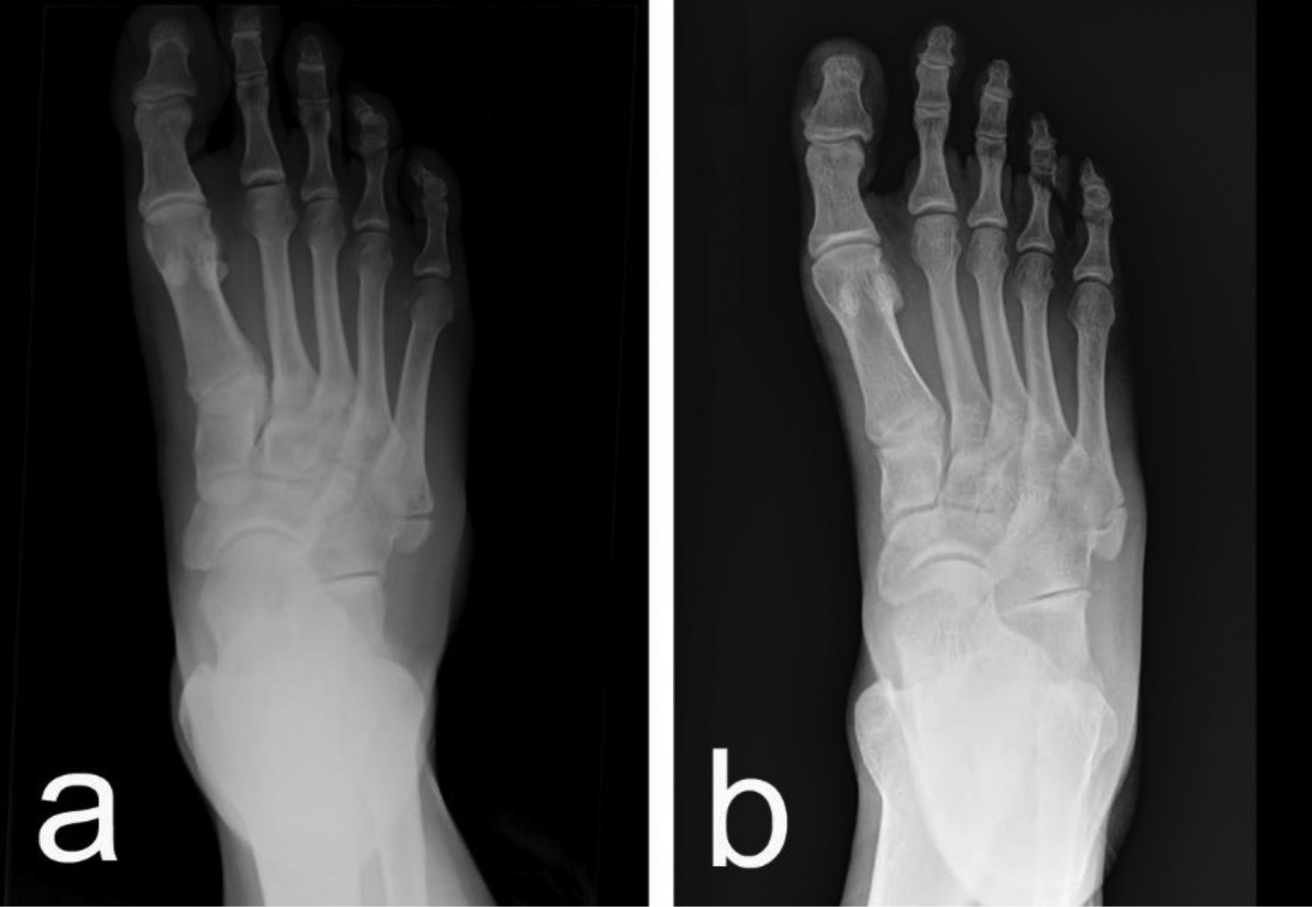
Plain radiographs of the two reported cases of symptomatic os vesalianum pedis (OVP). (a) Radiograph of the first patient, a 20-year-old male. (b) Radiograph of the second patient, a 43-year-old female. Both images demonstrate a well-corticated ossicle with smooth, rounded margins located proximal to the base of the fifth metatarsal
